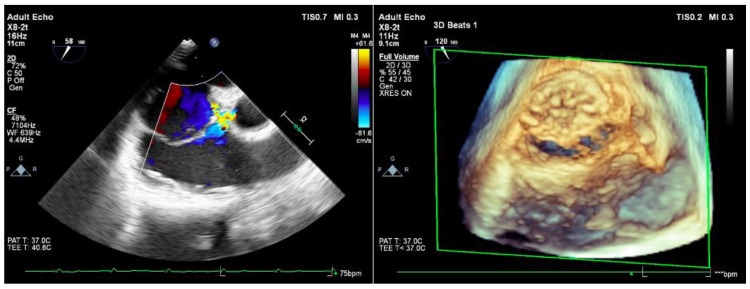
Postoperative transesophageal echocardiographic aspect.
